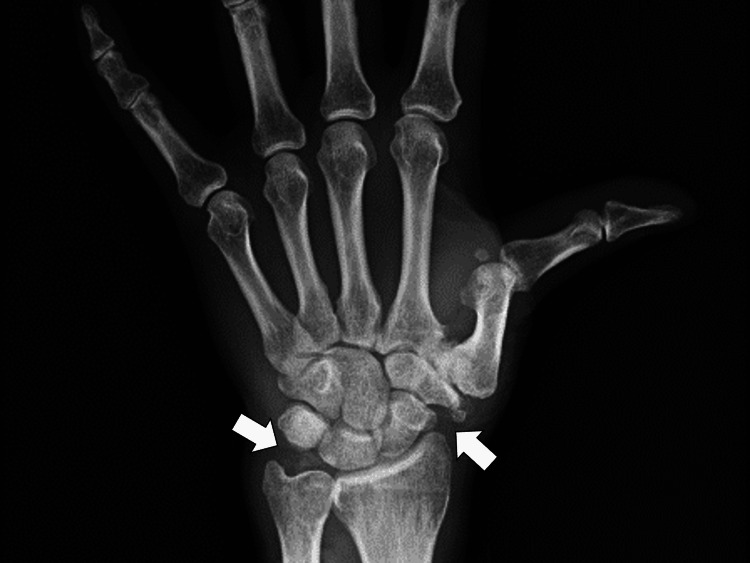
X-ray showing calcified deposits in the left wrist joint
